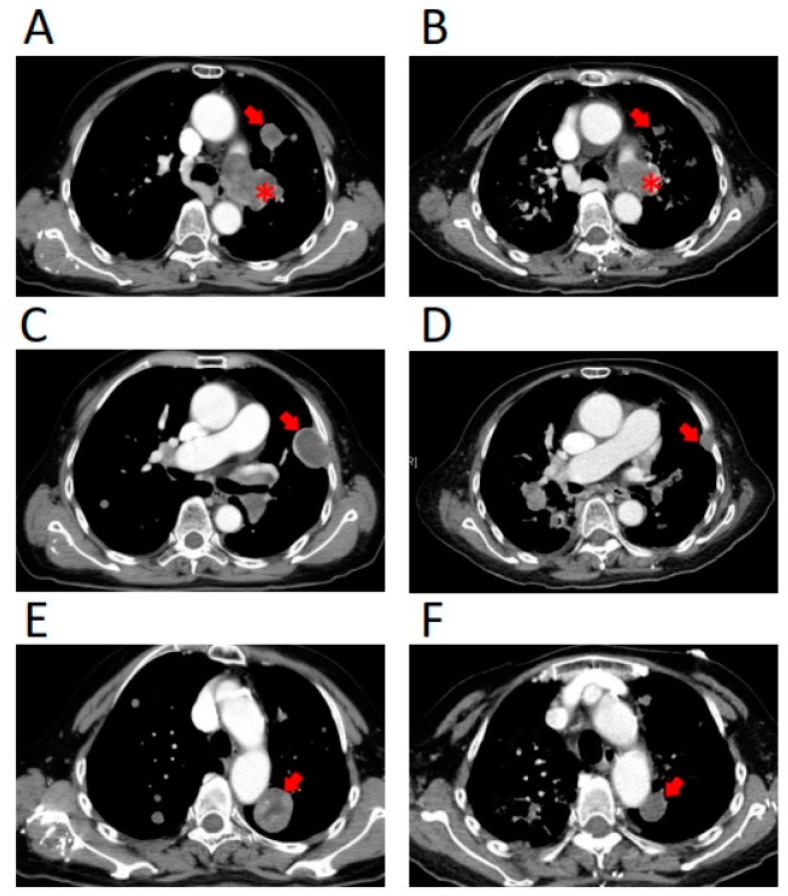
CT scan of the chest revealed a size reduction of tumors before (A,C,E) and after olaparib treatment (B,D,F). The asterisk (*) indicates a primary tumor, while the arrow symbol (→) indicates metastatic tumors. The primary tumor (*) decreased in diameter from 4.8 cm (A) to 3.0 cm (B) before and after olaparib treatment, respectively. The metastatic tumors of the lung (→) decreased in diameter from 2.0 cm (A), 3.4 cm (C), and 3.2 cm (E) to 0.8 cm (B), 1.6 cm (D), and 2.3 cm (F) before and after olaparib treatment, respectively. Partial response was achieved according to response evaluation criteria in solid tumors (RECIST) criteria.
